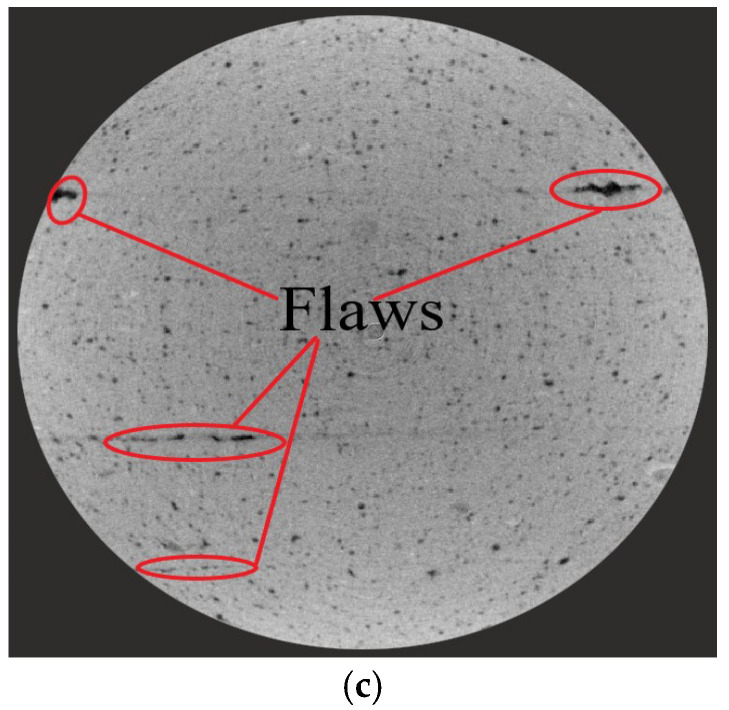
CT scanning images of the sample in different printing directions (1.1×). (a) Printing X-direction (b) Printing Y-direction (c) Printing Z-direction.
